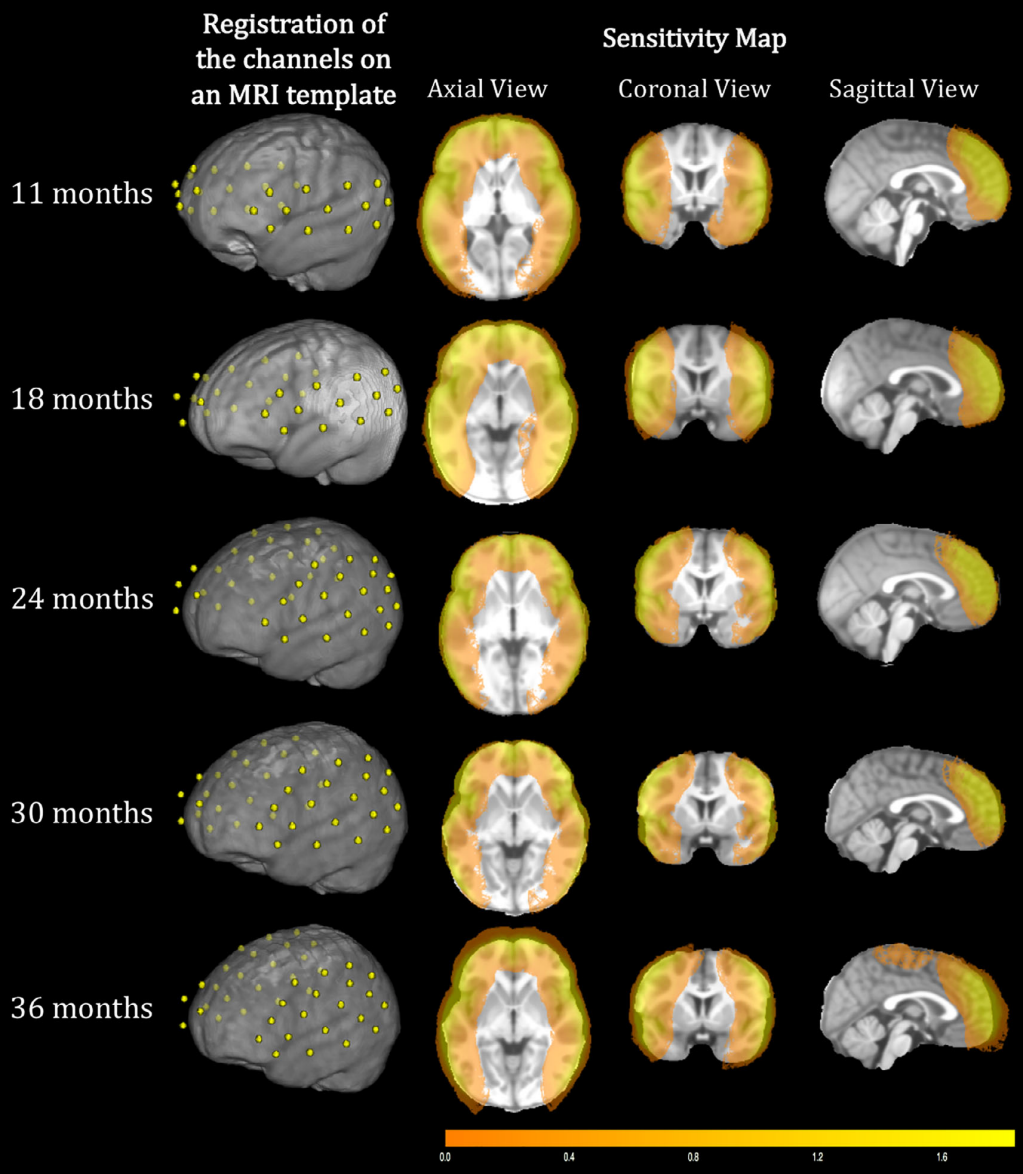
Registration of the channels on MRI templates and light attenuation changes in brain regions covered by the fNIRS array at every age
